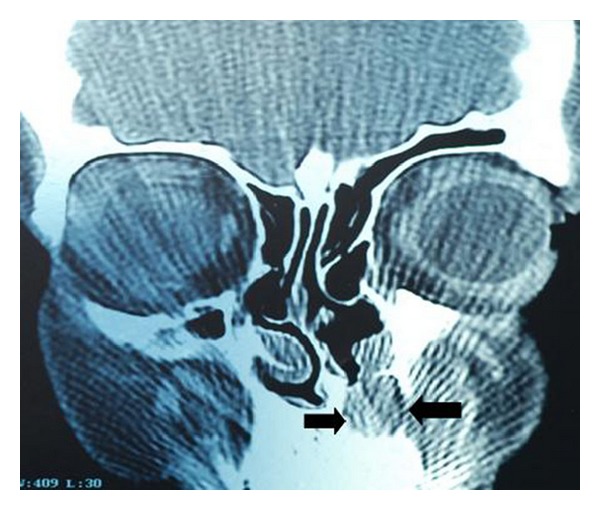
Coronal CT image showed a well-defined 1.5 cm diameter lesion in nasolabial fold.
